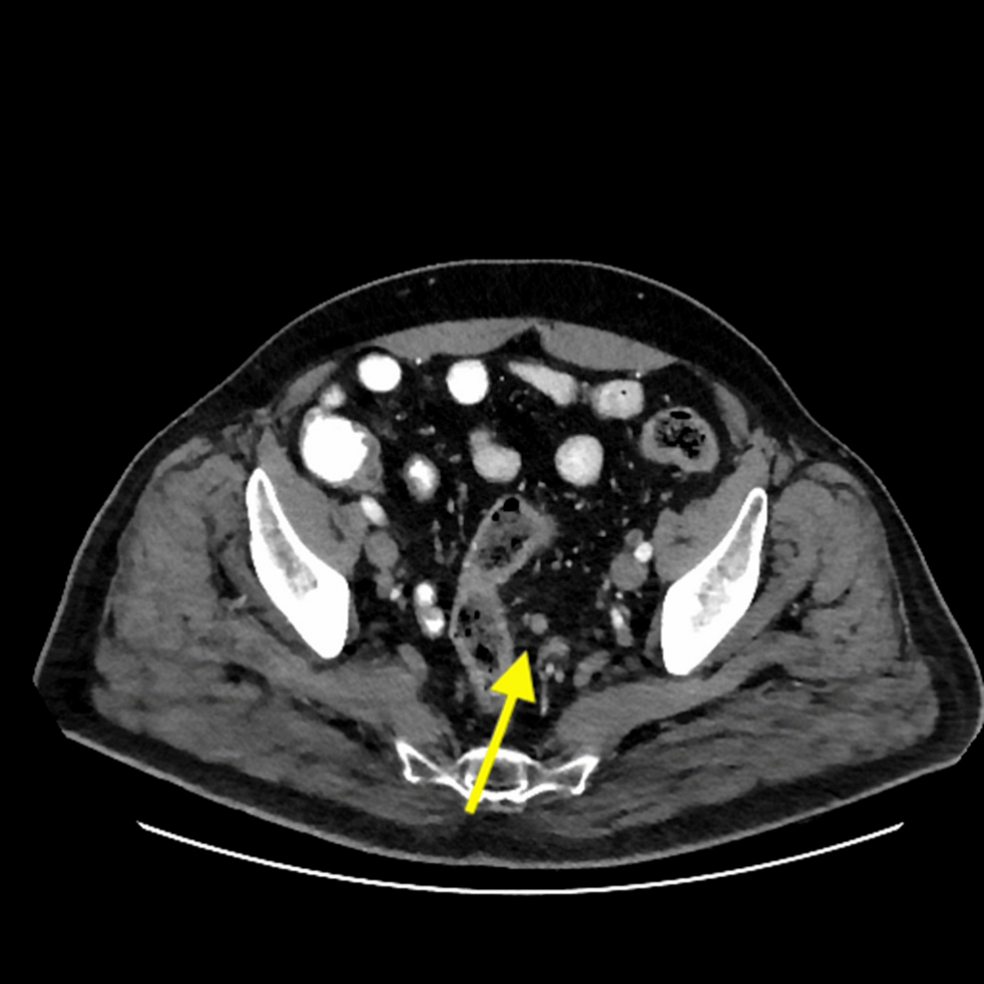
Computed tomography (CT) scan of the abdomen showing a prominent perirectal lymph node.
